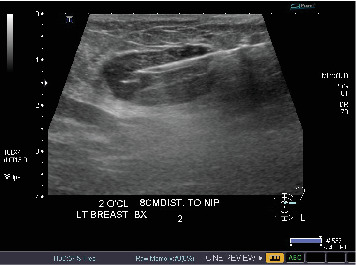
Ultrasound-guided biopsy of the mass in the left upper outer quadrant was performed. The above image shows the biopsy device with a needle tip in the mass.
